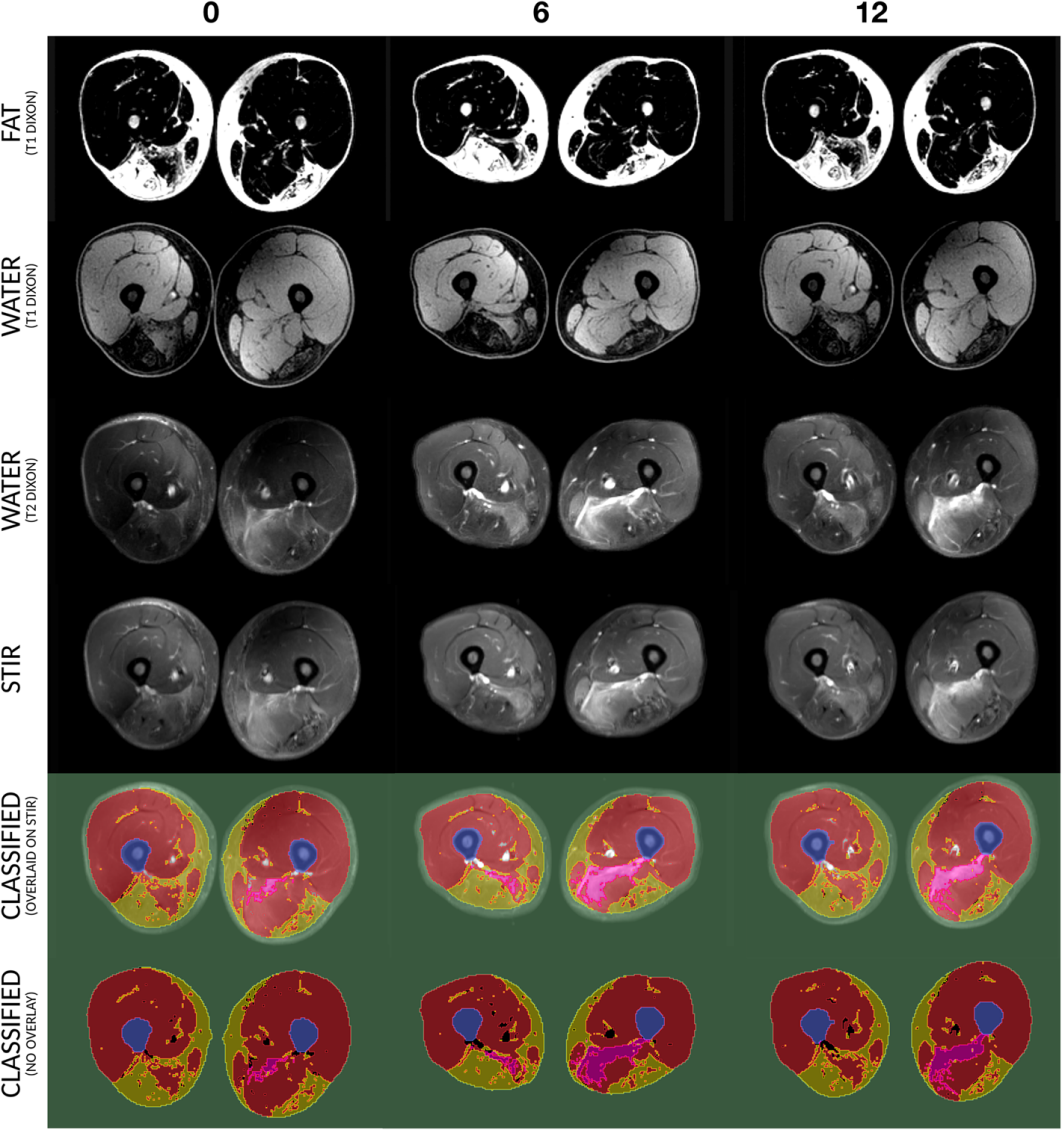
Vector Classification Example: From top, fat (T1 DIXON), water (T1 DIXON), water (T2 DIXON), and STIR images are shown from the same anatomical locations. Note the overlap between T2-weighted water measures and STIR+ signal. The vector segmentation overlaid on the STIR images follows, demonstrating the close correspondence between classes of air and subcutaneous tissue (green), bone (blue), muscle (red), fat (yellow), and STIR+ signal (pink). In the raw segmentation without overlay (bottom), black pixels are edited large vessels
